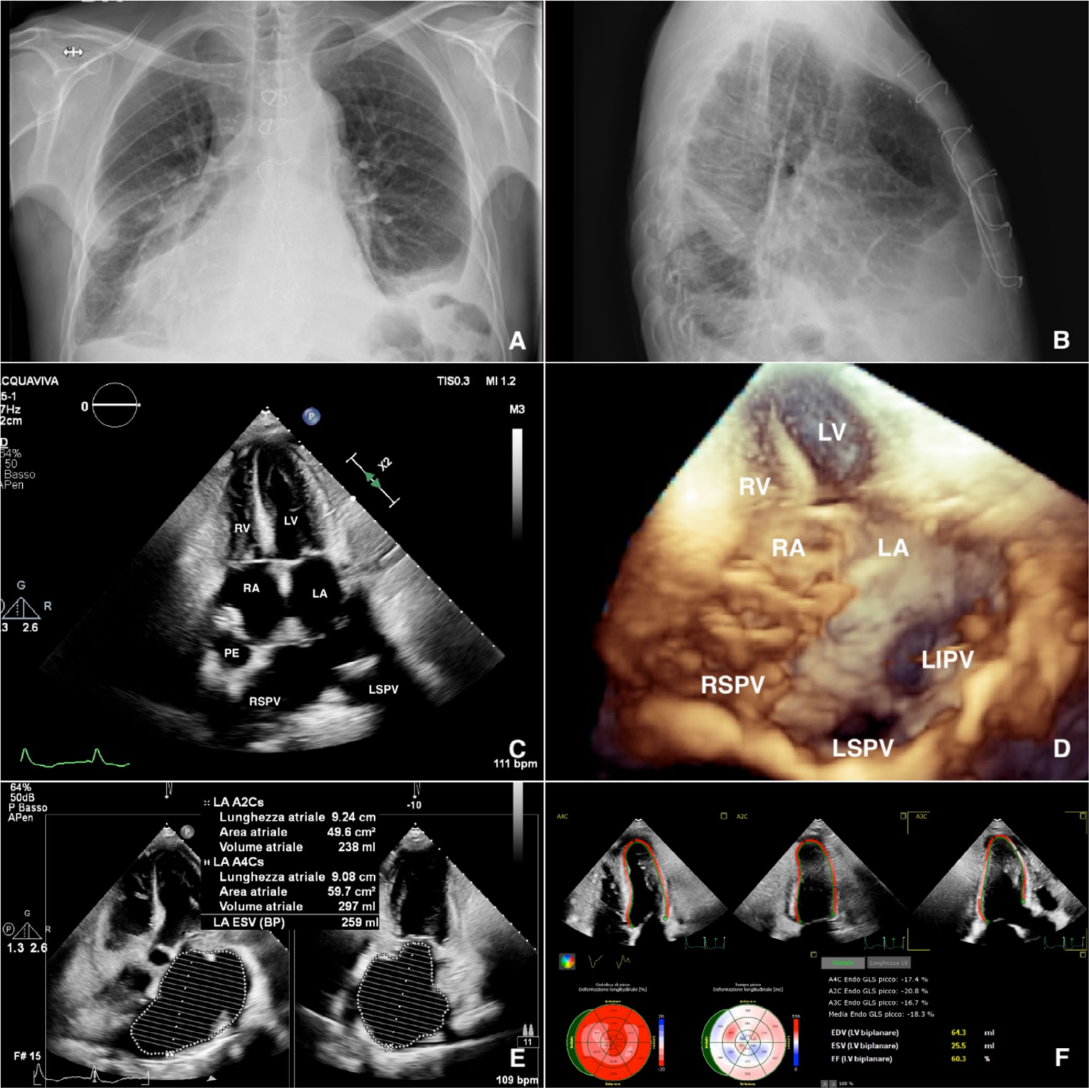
Post‐operative imaging: Chest X ray (A, B); Transthoracic echocardiogram 2D apical 4‐chamber (C) and real time 3D (D), biplane end‐systolic LA volume (E), global longitudinal strain of −18% and biplane left ventricular ejection fraction of 60% (F). LA, left atrium; LIPV, left inferior pulmonary vein; LSPV, left superior pulmonary vein; LV, left ventricle; PE, pericardial effusion; RA, right atrium; RSPV, right superior pulmonary vein; RV, right ventricle.
